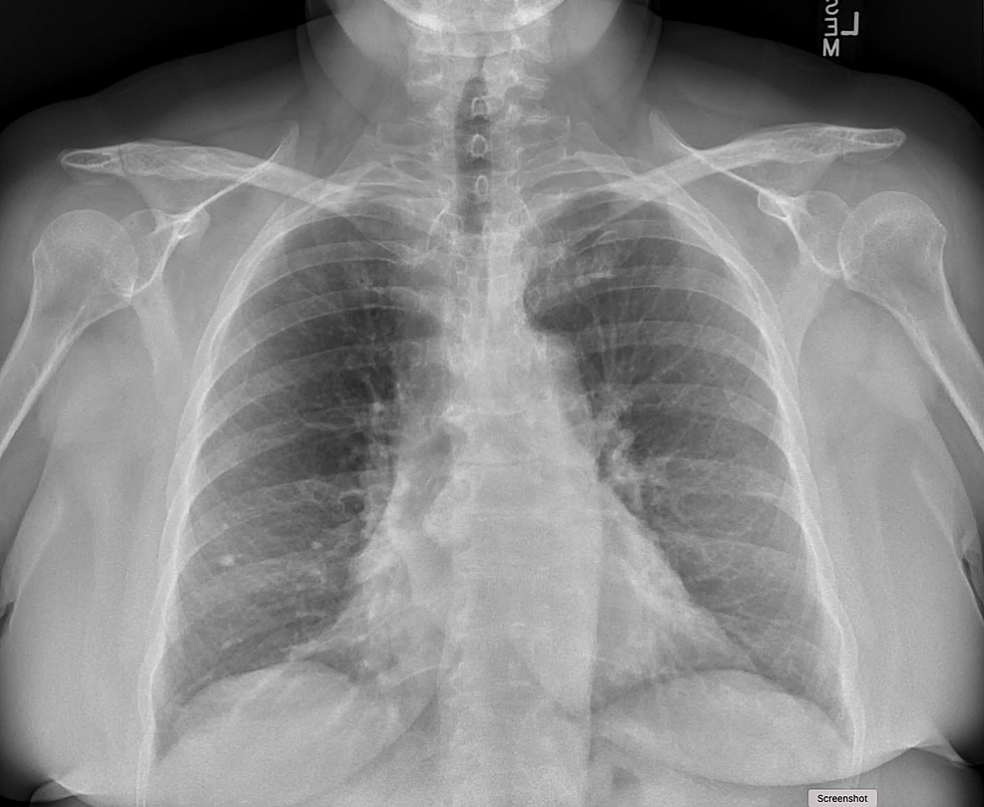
Chest X-ray following therapy showing complete clearing of bilateral infiltrates
